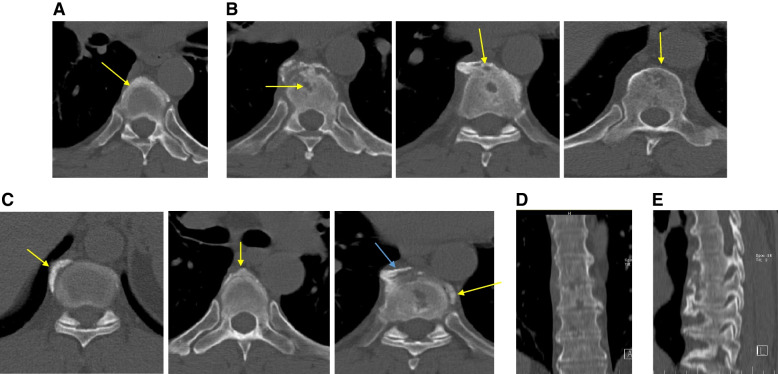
Sagittal and coronal CT of thoracic spines. Coronal view shows markedly cortical thickening (hyperostosis) of thoracic vertebrae (A) and multiple cortical erosions (B). Severely thickened ossification of anterolateral spinal ligament along with large osteophytes *without disk space involvement* suggestive of DISH (C). AP view showing relative symmetrical non-marginal syndesmophytes (D). Sagittal view shows thick flowing osteophytes consistent with DISH (E). CT, computed tomography; AP, anteroposterior; DISH, diffuse idiopathic skeletal hyperostosis. All radiographic images were screenshotted from the compact discs.
